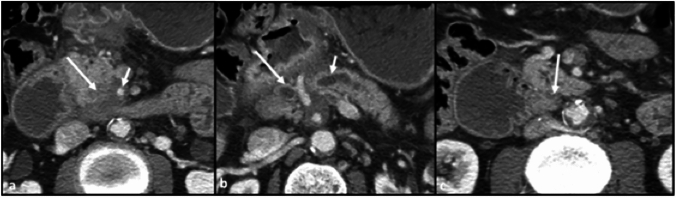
Axial CT sections with contrast in the portal venous phase. a Ill-defined, hypodense mass in the posterior pancreatic head (long arrow), which encases the superior mesenteric artery (short arrow). b Dilatation of the common bile duct (long arrow) and the pancreatic duct (short arrow), indicating obstruction at the level of the ampulla. c Infiltration of disease into the 3rd segment of the duodenum (arrow)
